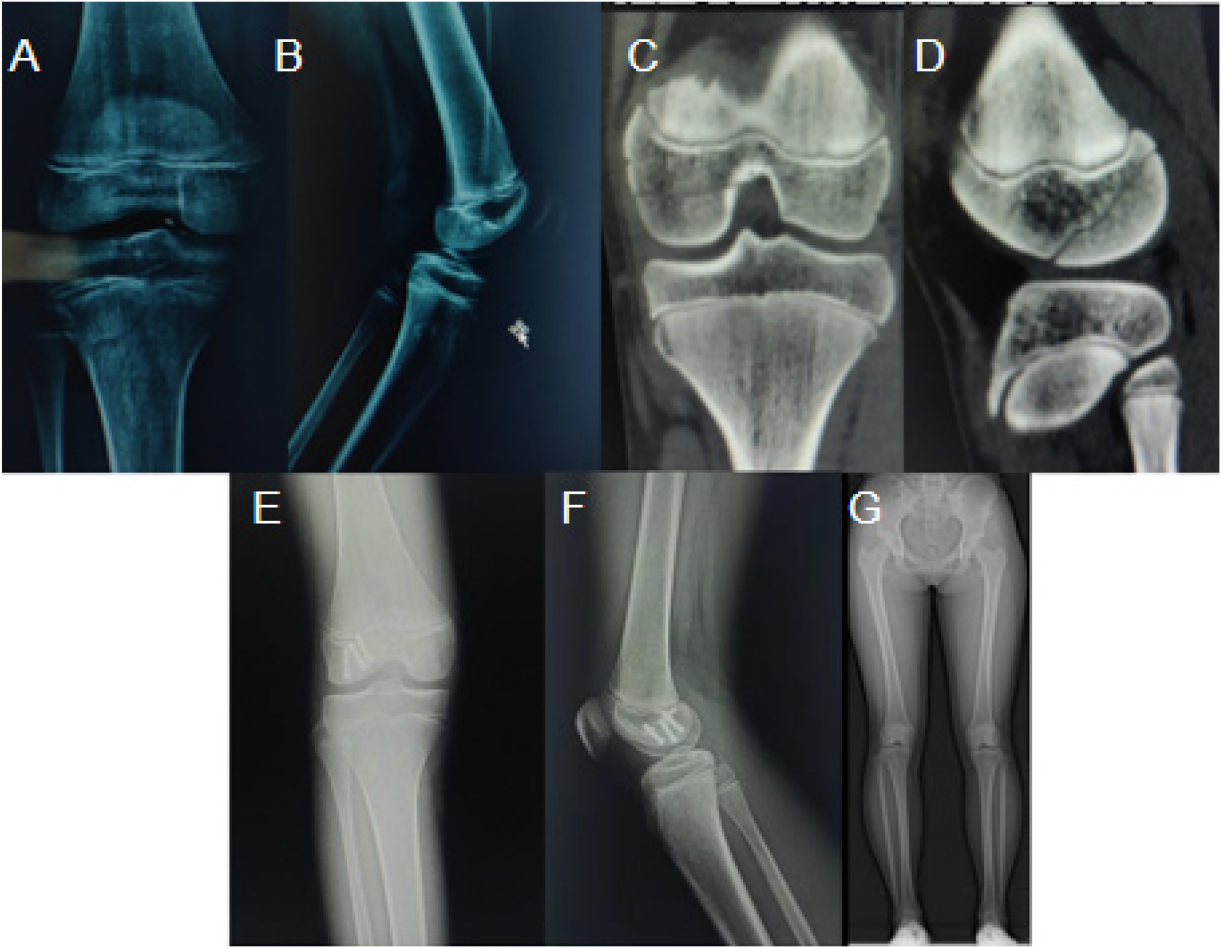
A case of a 12-year-old girl with a right lateral femoral condyle fracture from a car accident. (A, B) Preoperative X-rays showing the break; (C, D) preoperative CT images for detailed conditions; (E, F) Postoperative X-rays showing bone healing; (G) Full-length X-ray of both lower limbs three years post-surgery.
